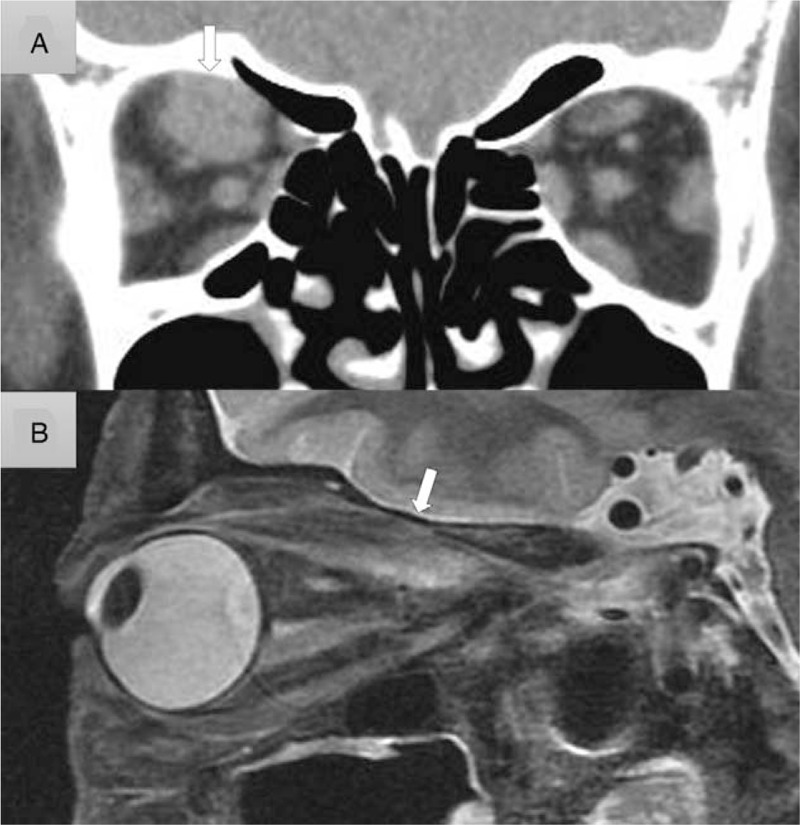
Series of clinical imaging scans showing the development of compressive optic neuropathy (CON). A. Coronal imaging of a computed tomography (CT) scan shows the distinguished right superior levator / superior rectus (SL/SR) muscle complex enlargement (arrow) that caused CON. B. Sagittal imaging of the MRI scan shows that the belly of enlarged SL/SR muscle complex (arrow) is proximate to the orbital apex and is apparently compressing the optic nerve.
